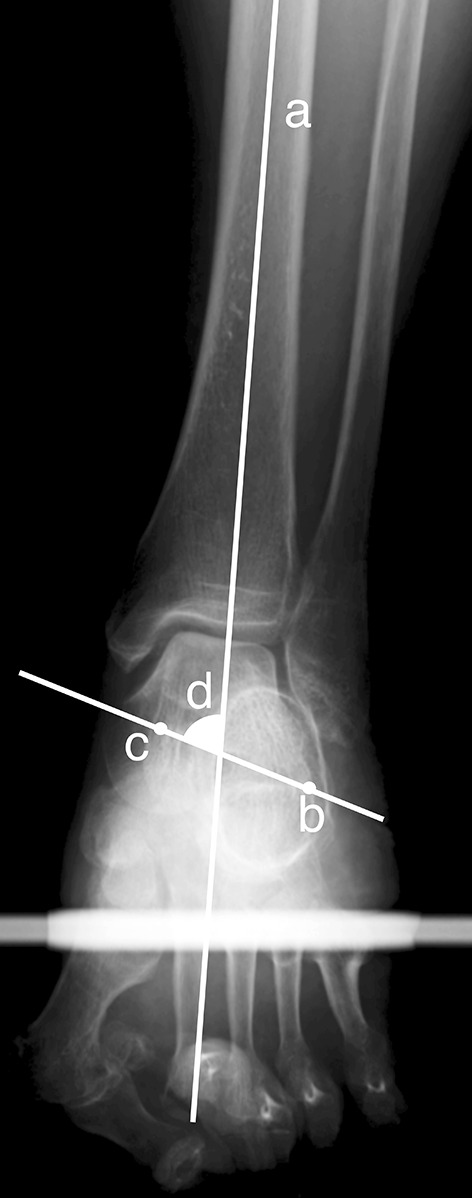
Hindfoot radiograph image. *Line*
